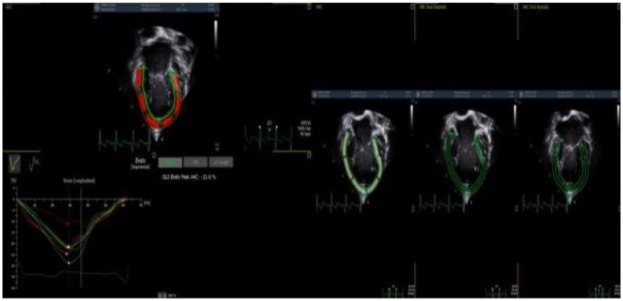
Global longitudinal strain (*G*LS) measured from an apical four-chamber view, tracing the endocardial border. Peak systolic longitudinal strain is recorded from all six segments of the myocardium and GLS is calculated as the average of all available segments.
